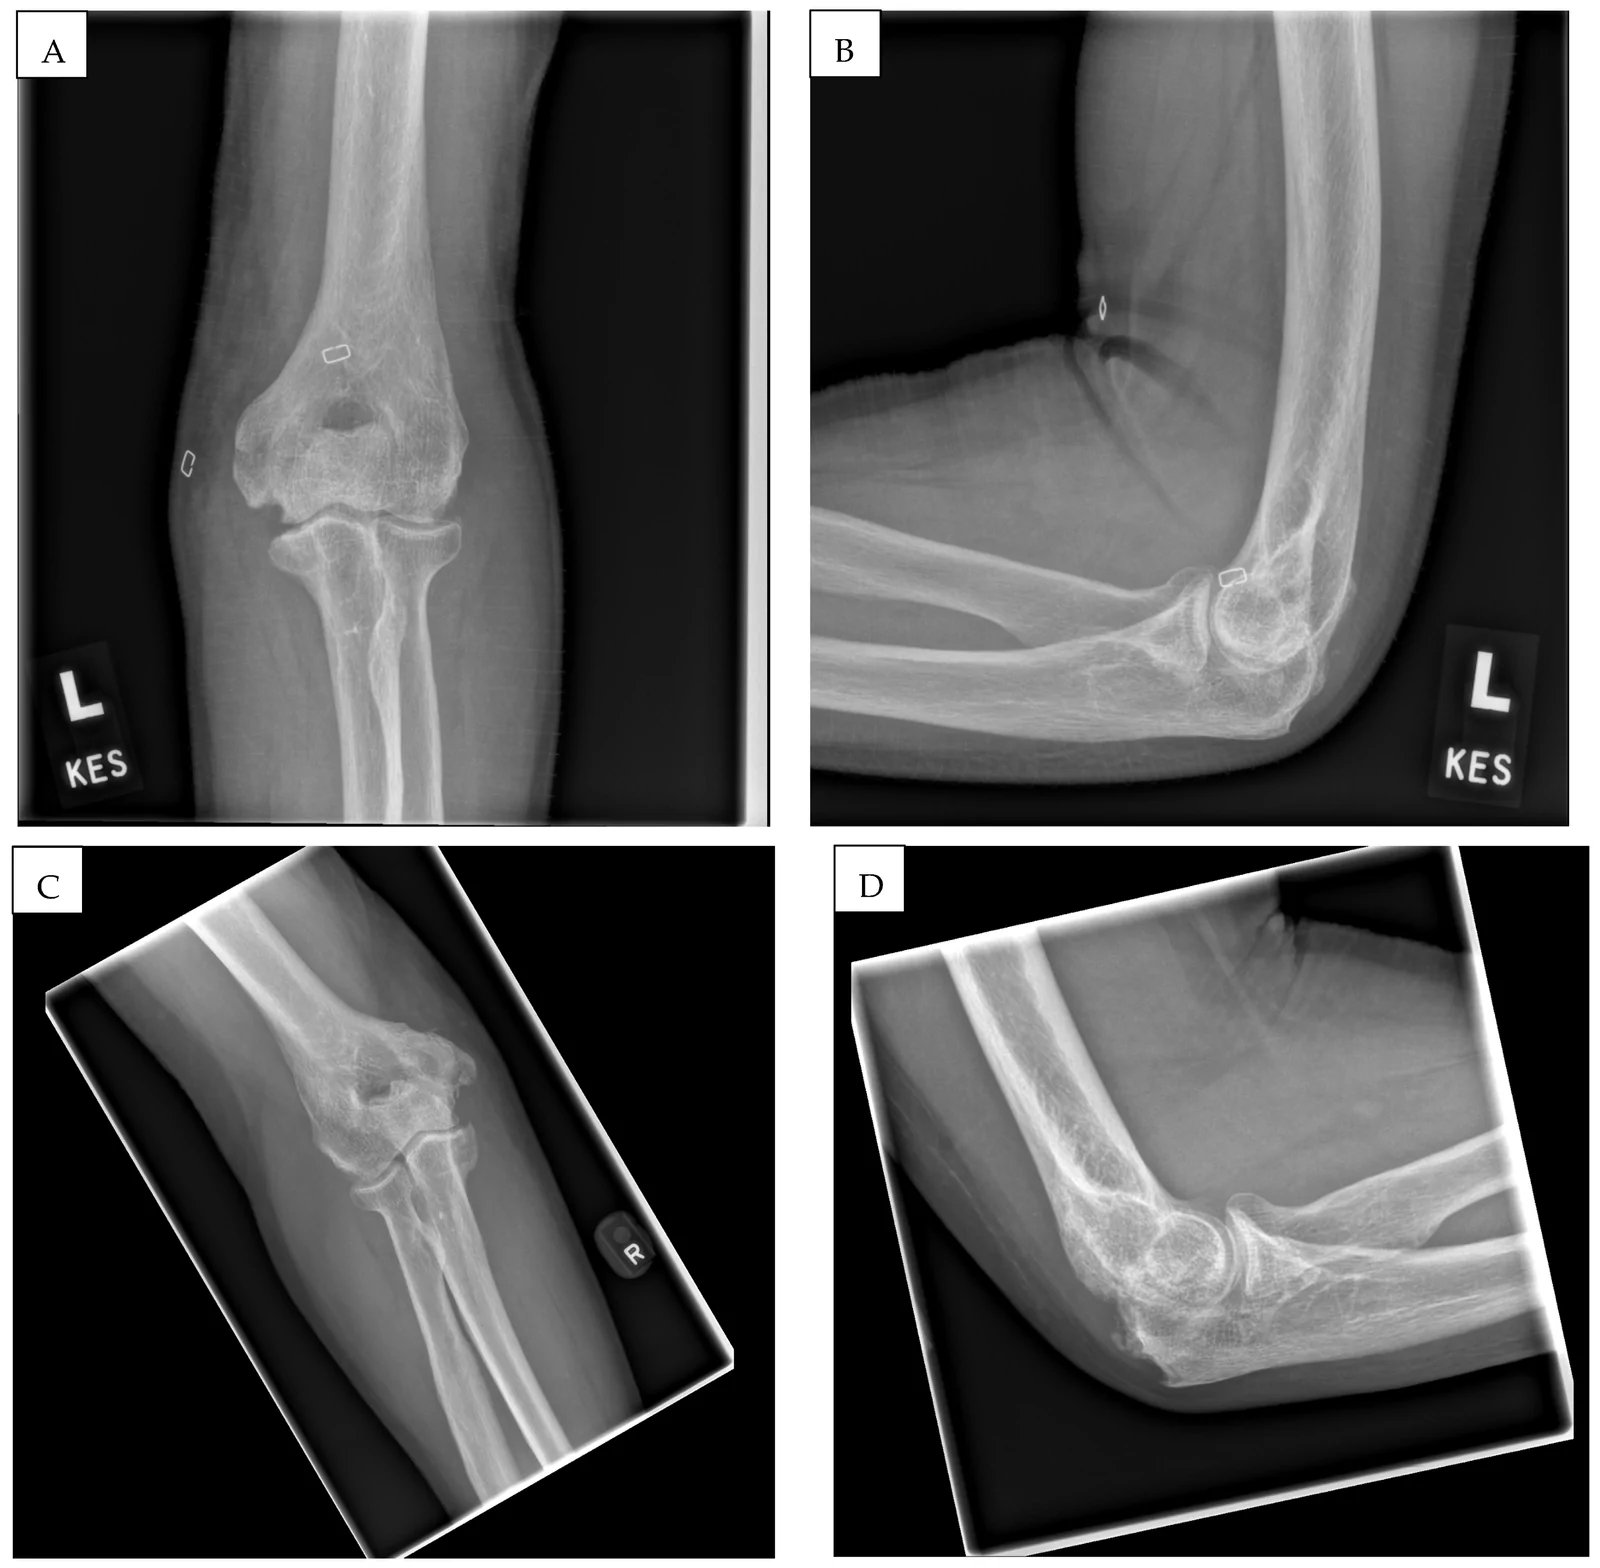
Postoperative (A,C) anterior to posterior (AP) radiographs of the left and right upper extremities, respectively. (B,D) Lateral radiographs of the left and right upper extremities, respectively. This figure demonstrates appropriate alignment, healing, and omission of abnormal bony overgrowth at final follow-up, bilaterally.
